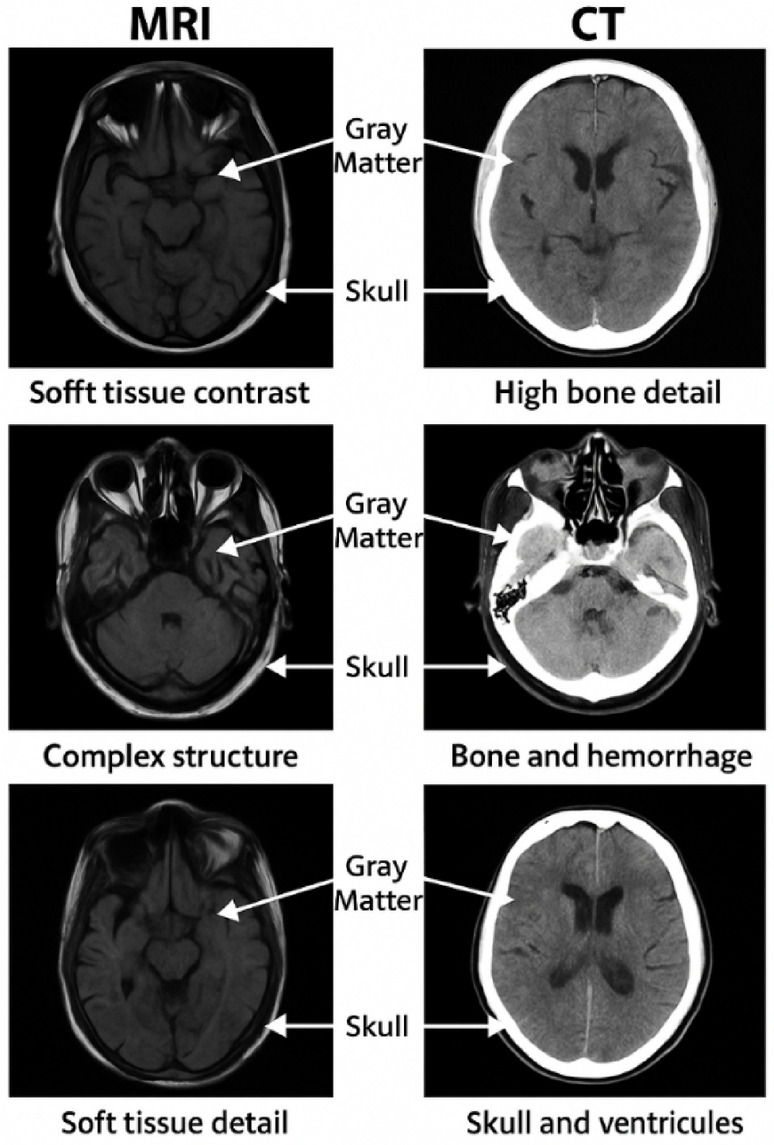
Representative head images from MRI (left column) and CT (right column) scans with annotated regions of diagnostic importance.
